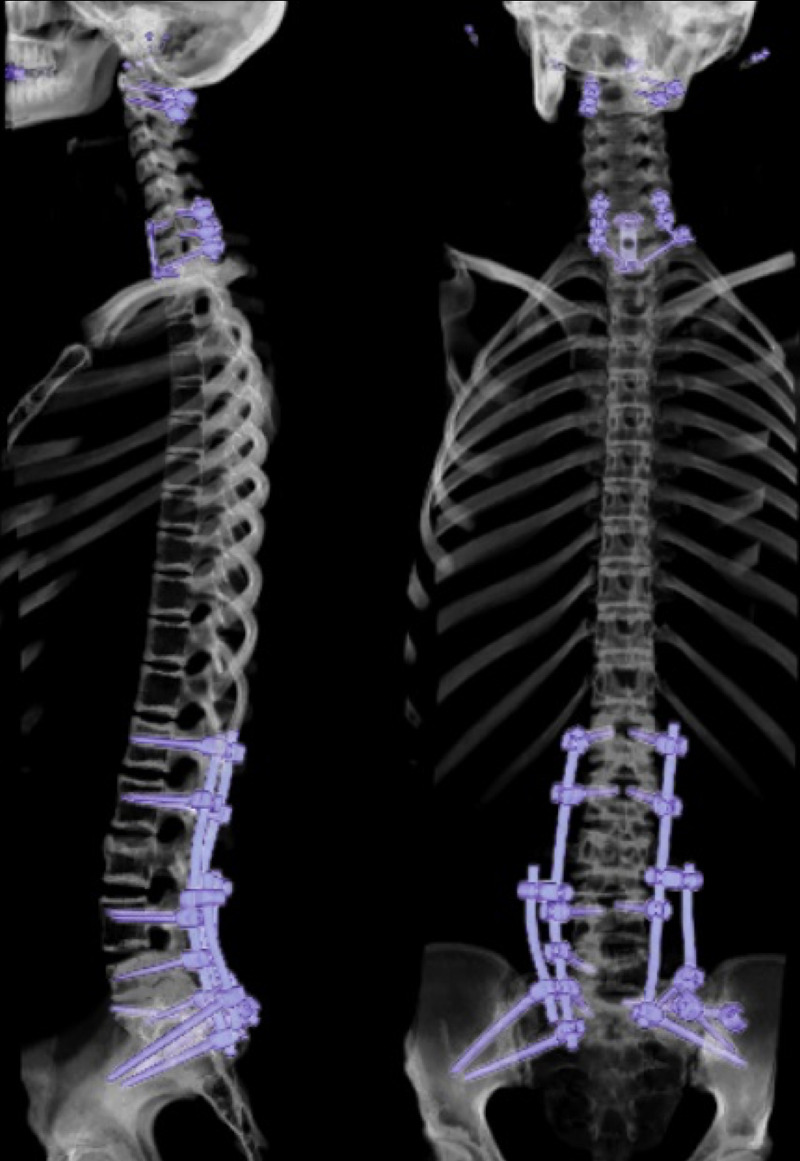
postoperative CT-3D imaging. C1 lateral mass screw combined with C2 pedicle screws, C6-T1 anterior stabilization with mini-plate screws and C6-C7 lateral mass and T1 transpedicular screws, Placement of bilateral transpedicular screws and rods was carried out at L1, L2 and L4 and on the left at L5. At S1 and S2 sacrum-alar and iliac screws and percutaneous sacral screws were also inserted
